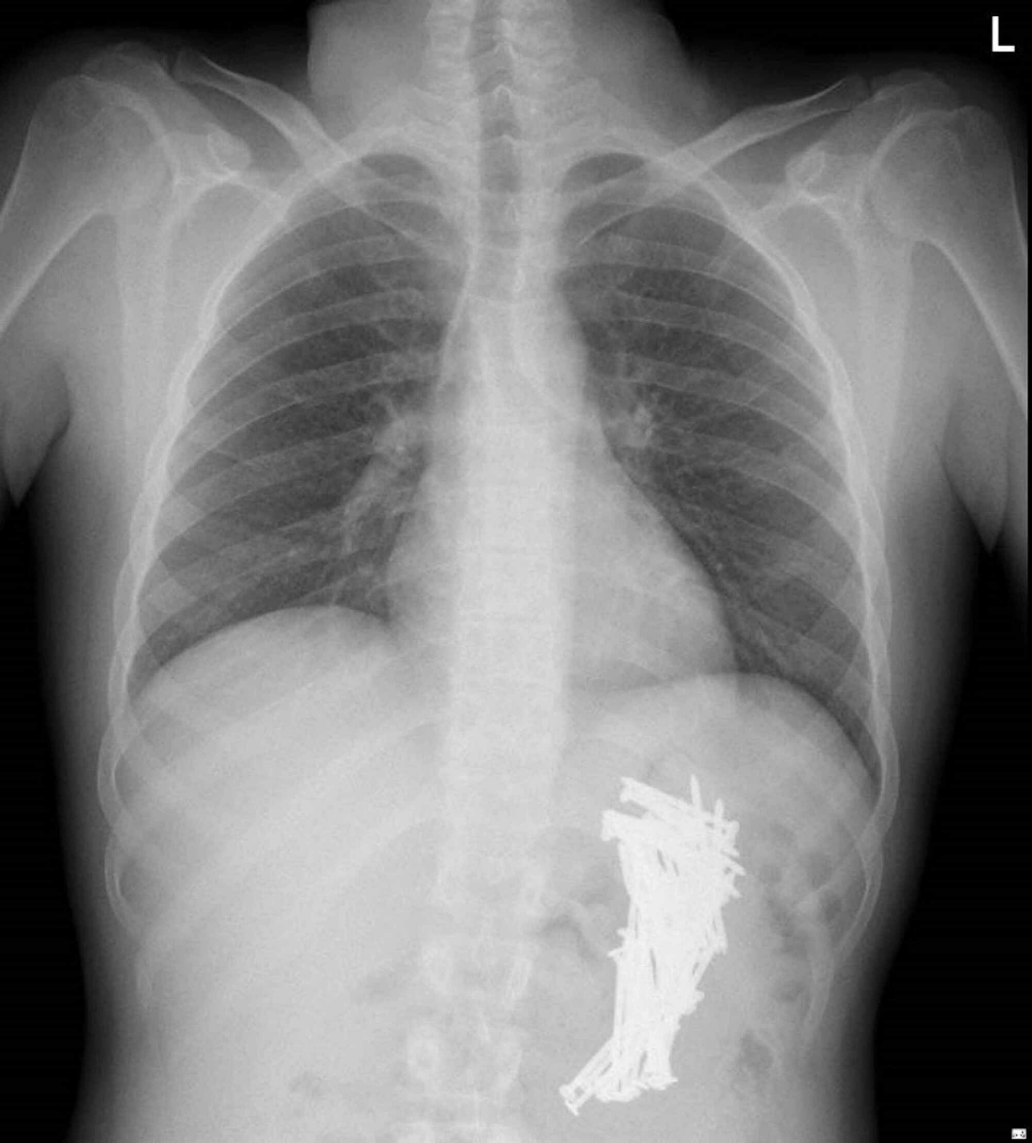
Chest X-ray showing multiple metallic nails within the stomach, with no evidence of air under the diaphragm
